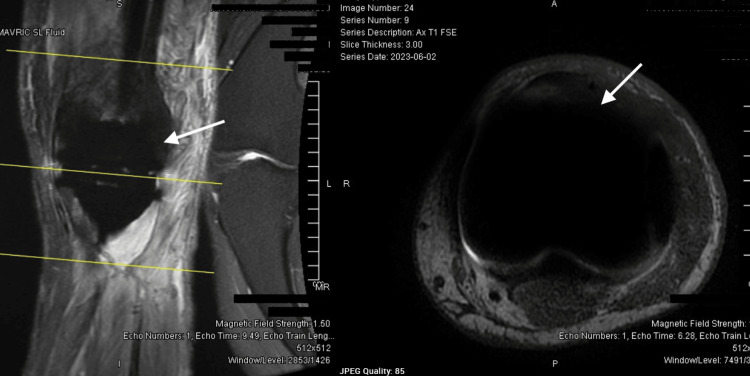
MRI of the left knee post-reconstructive surgery A: Complete excision of the mass from the left knee. Notable post-surgical changes include the appearance of the surgical site and surrounding edema B: Post-reconstructive surgery view of the left knee highlighting the reconstructed structures and the surrounding soft tissues MRI: magnetic resonance imaging
